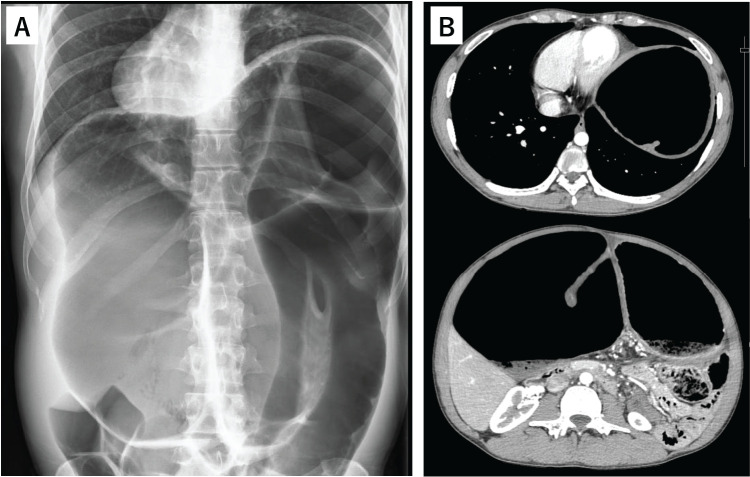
Imaging findings at initial presentation. (A) Abdominal radiography shows diaphragm elevation, with rightward displacement of the heart. (B) Enhanced CT shows marked colonic dilatation, with rightward displacement of the heart.
